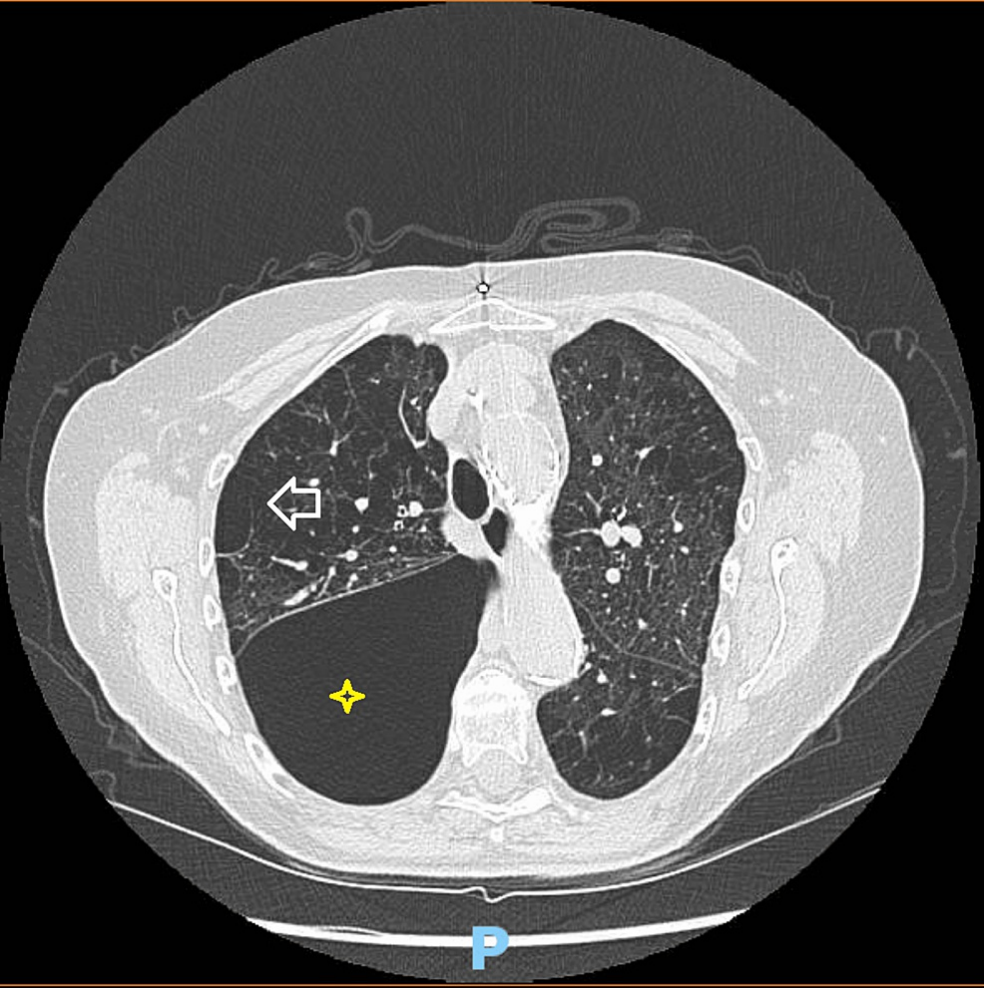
CT chest showing a pneumothorax (asterisk) measuring 11.9 x 8.9 cm with extensive emphysema (arrow) of the bilateral lung parenchyma.
